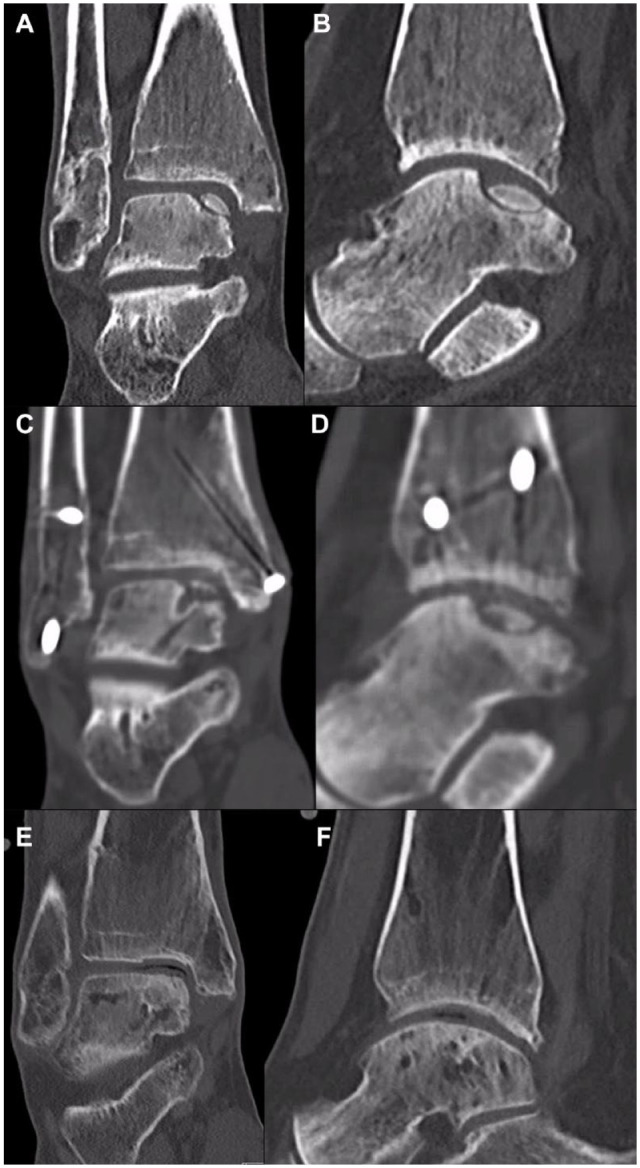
Case 4. Pre-operative CT with coronal (A) and sagittal (B) views, showing a pseudoarthrosis of the distal fibula and an osteochondral fragment on the posterior-medial talar dome. The fragment measured anteriorposteriorly: 15 mm, medial-laterally 10 mm, and depth: 6 mm. The 8.5 months post-operatively after sustaining an inversion injury the CT with coronal (C) and sagittal (D) views showed a consolidated medial malleolus osteotomy, consolidated pseudoarthrosis repair of the distal fibula but a non-union of the osteochondral fragment without fragment displacement or signs of hardware complications. Hereafter, the patient underwent a medial TOPIC procedure. The results were satisfactory with a good incorporation of the graft and osteotomy union at the 3-year follow-up as outlined on the 3-year post-operative CT with coronal (E) and sagittal (F) views.
